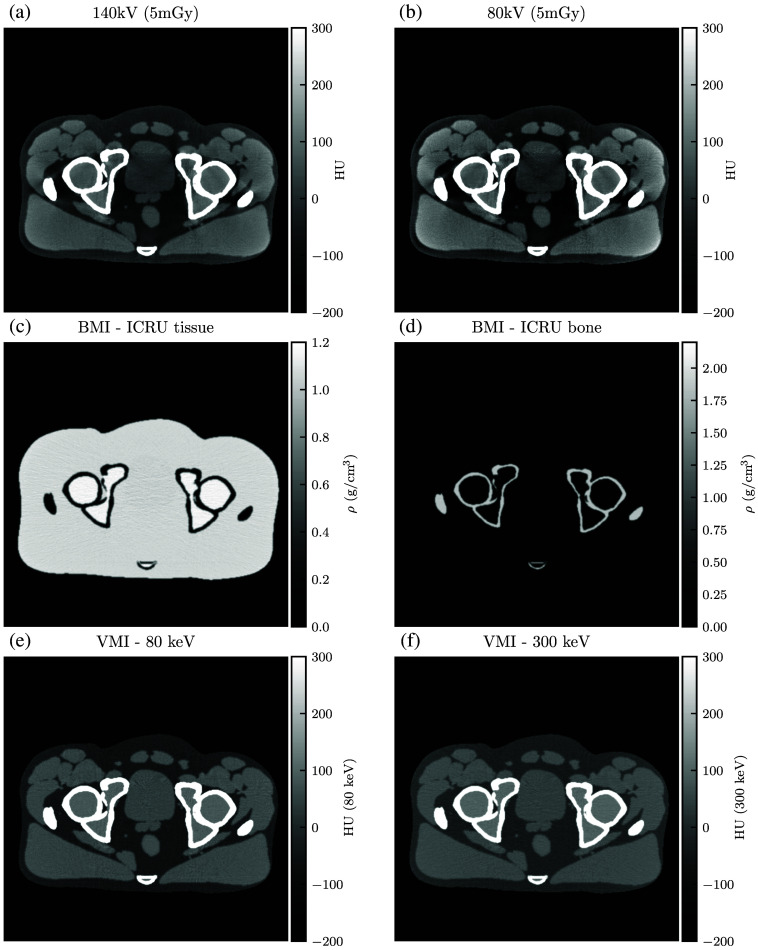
(a) and (b) Single-energy CT images, (c) and (d) BMIs, and (e) and (f) VMIs for the 140 kV-80 kV DE-CT acquisition of the pelvis with no hip replacement. The VMIs correct the beam hardening visible in the single-energy CT images.
